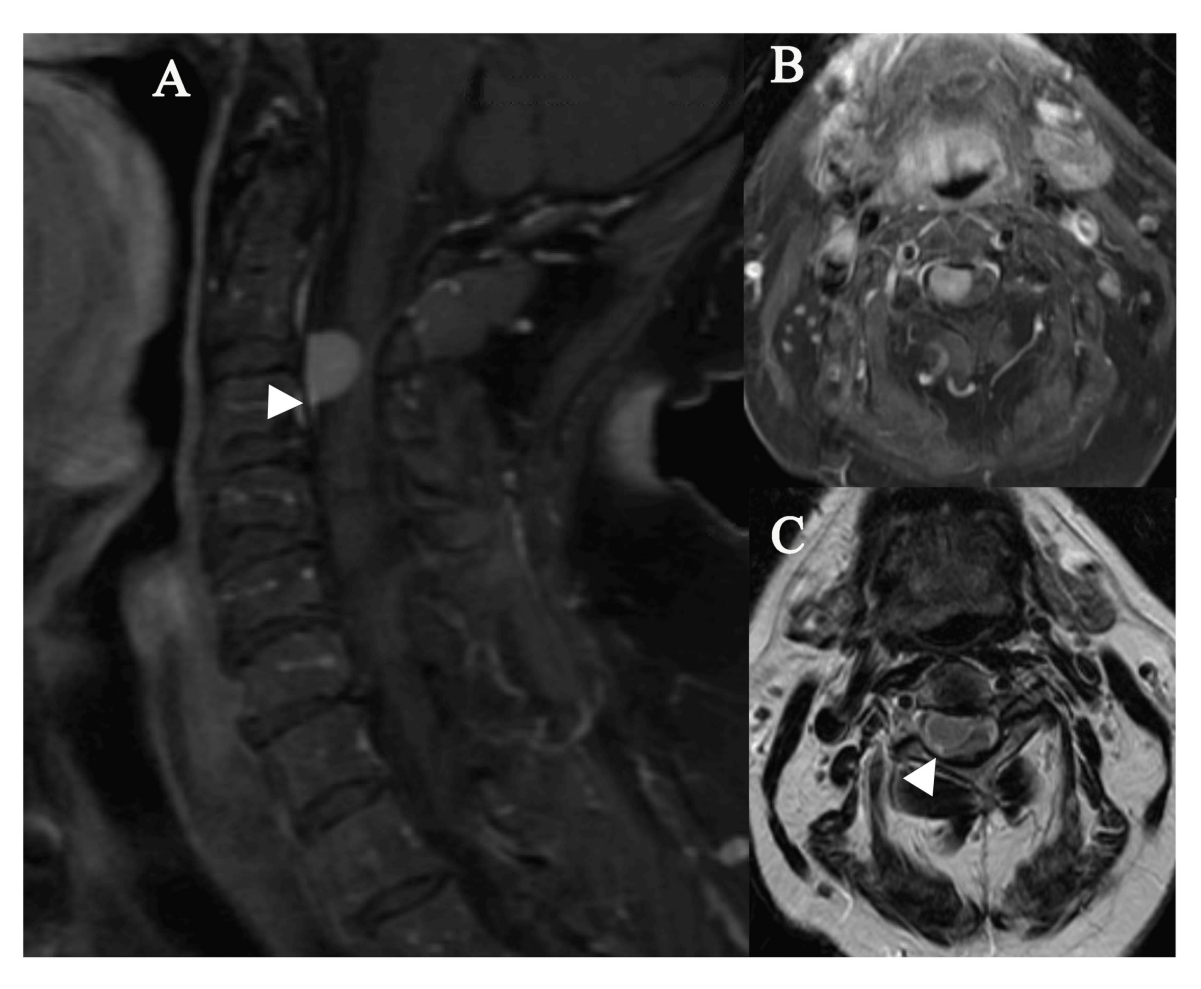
Preoperative MRI in multiple views (A) Sagittal post-gadolinium MRI demonstrating the ventral location of the tumor with significant canal compromise. The triangle denotes the dural tail, a pathognomonic feature of meningioma. (B) Axial post-gadolinium MRI demonstrating the ventral location and canal compromise. (C) Axial T2 sequence: the triangle denotes the border between the meningioma to the top left of the triangle and the spinal cord, which is to the bottom and right of the triangle. The spinal cord is significantly compressed and displaced dorsally and toward the patient's left.
